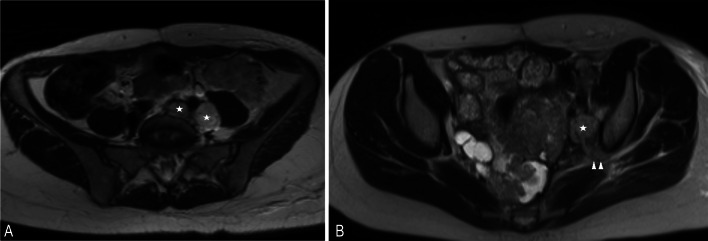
a, b Two different patients with biphasic malignant peritoneal mesothelioma. MRI shows (a) retroperitoneal (*) nodes and (b) left pelvic side wall lymph node (*). Note the disease extension into the left sciatic notch (b, arrow heads)
